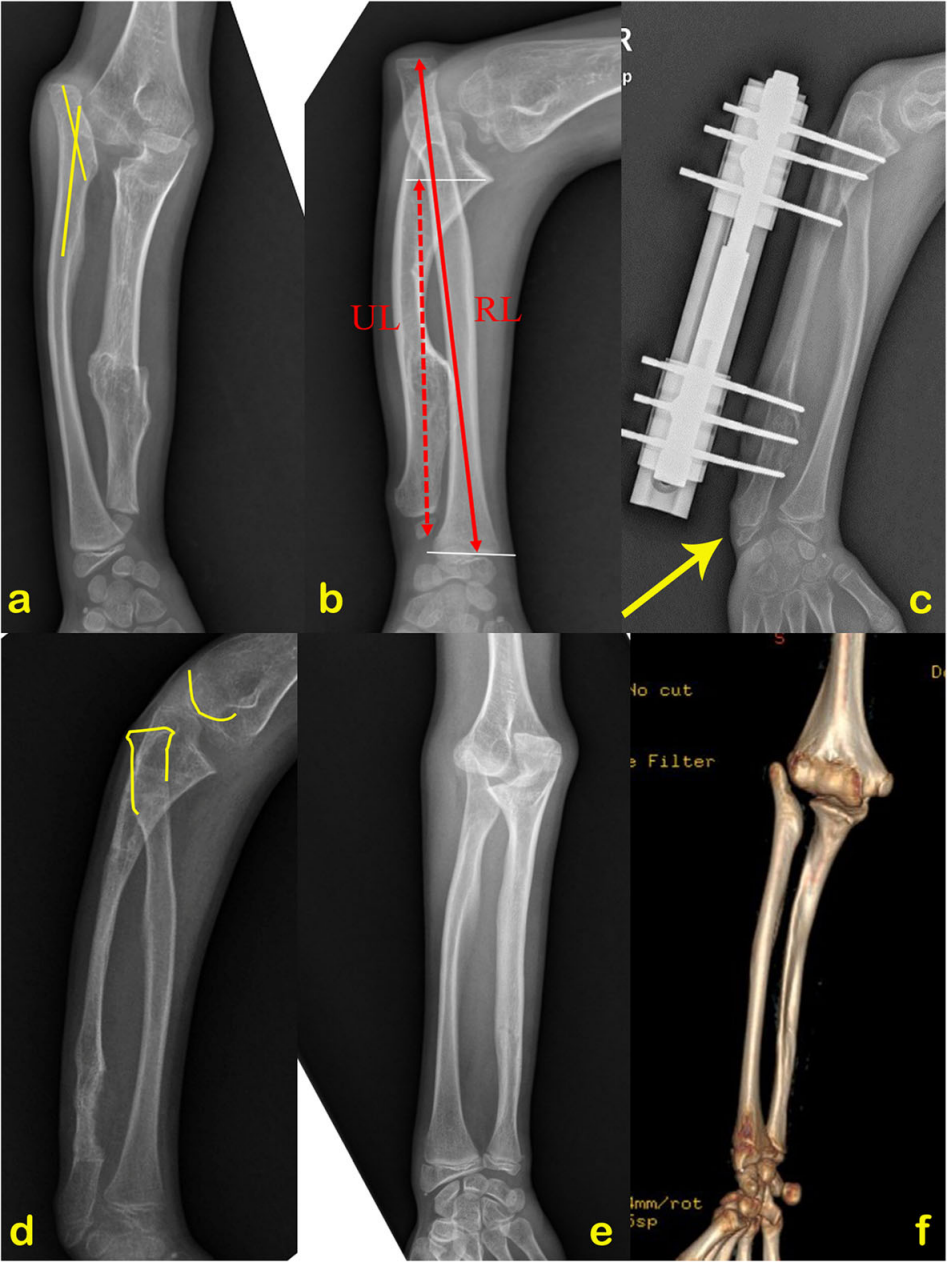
a Type IIb deformity in a patient with HMO, adaptive lateral angulation of the proximal radius due to the long-standing dislocation of the radial head. b In the lateral view, radial length (RL) is the distance from the center of the proximal radial physis to the center of the distal radial physis. Ulnar length (UL) is the distance between the trochlear and the ulnar styloid on the longitude axis of the ulna; ulnar shortening was determined by the RL minus the UL. c, d The ulna was overlengthened for 15 mm, and the radial head was not reduced by gradual ulnar distraction. e, f The patient came back 3.1 years after surgery, and computed tomography (CT) was performed, demonstrating the posterolateral displacement of the radial head, but the distal radioulnar joint was remolding well
